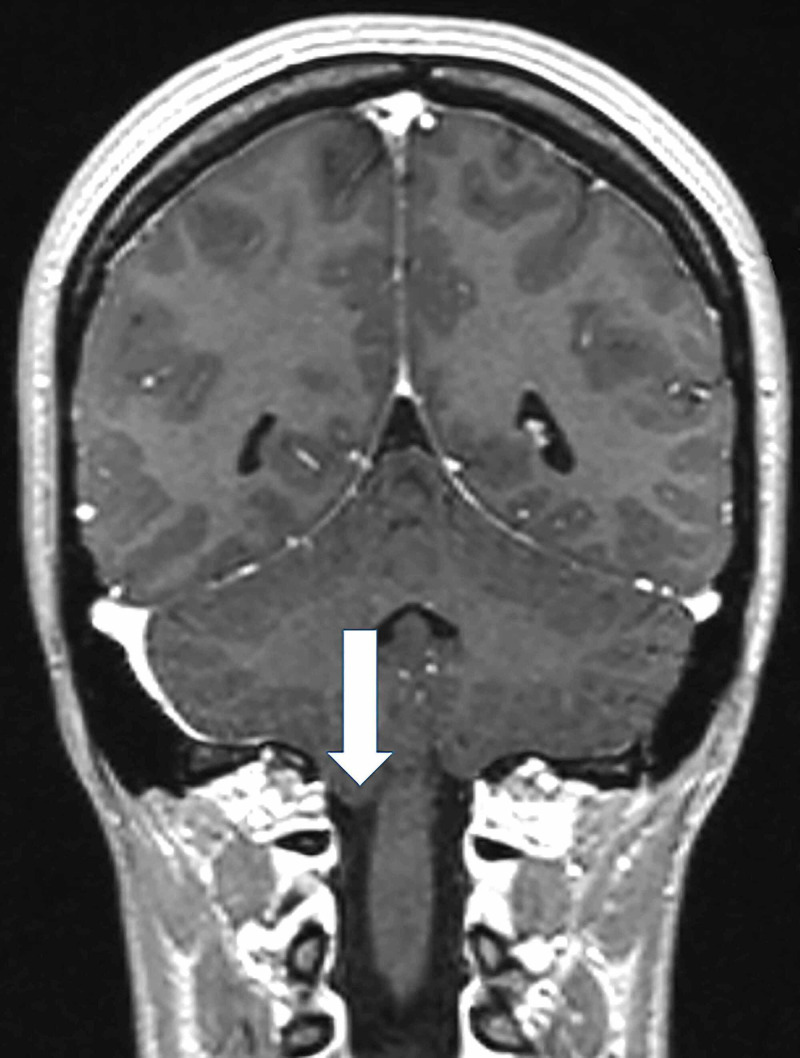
Coronal brain MRI. Coronal brain MRI showing cerebellar tonsil herniation (arrow) through the foramen magnum.
